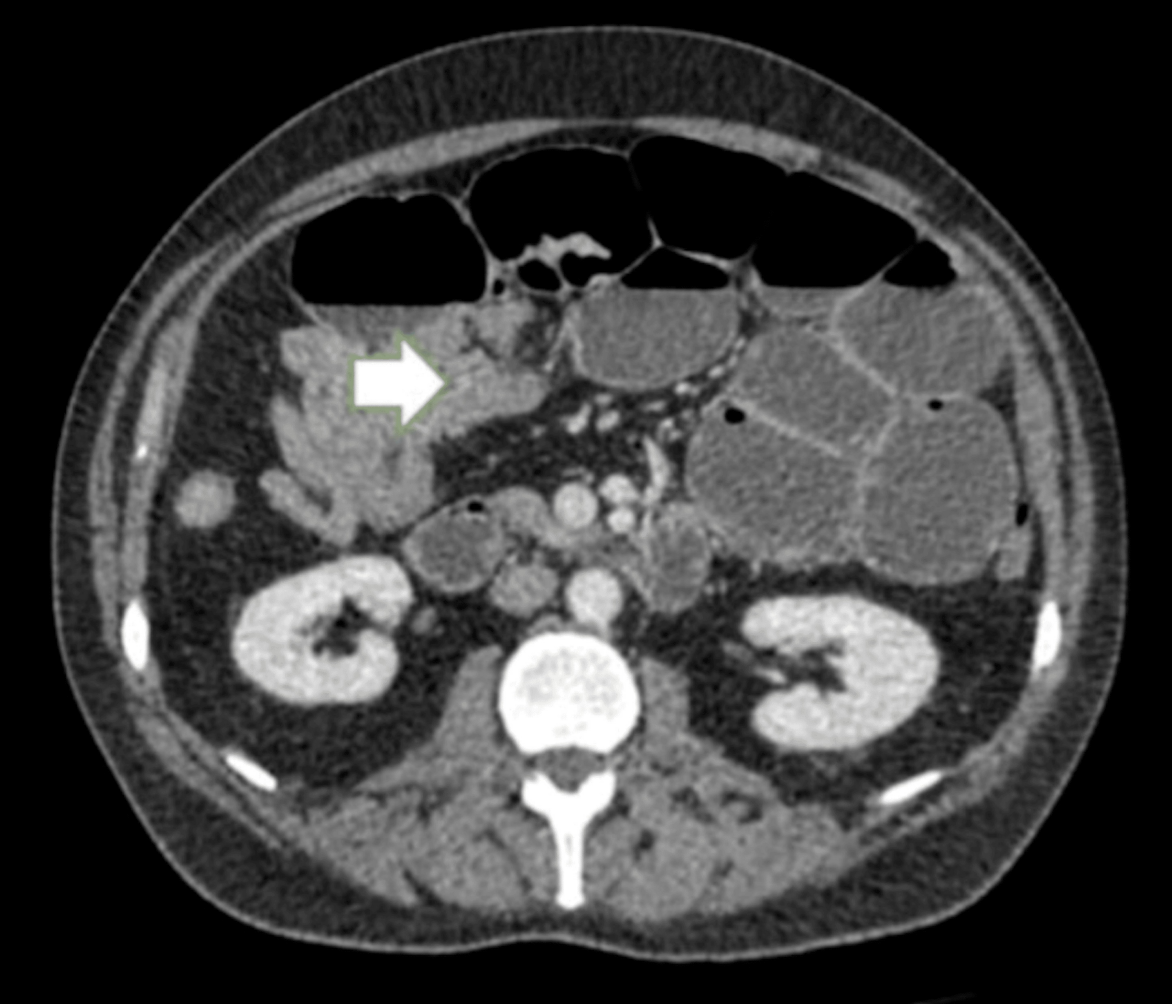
Contrast-enhanced CT abdomen and pelvis (axial section) showing jejunal obstruction (white arrow)
